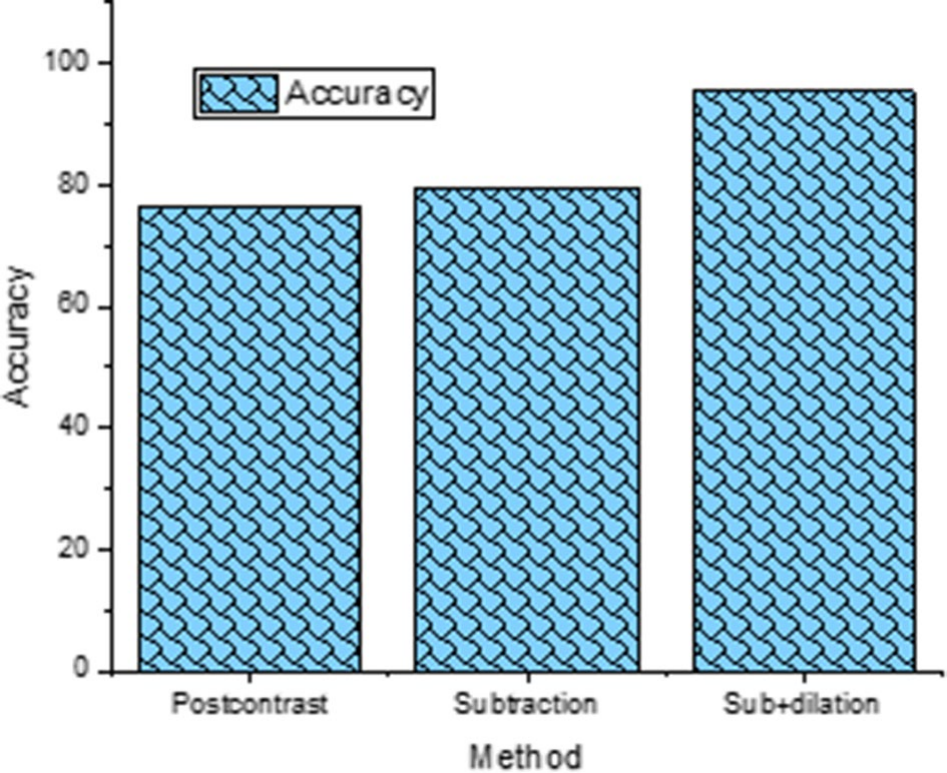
Accuracy for MRI texture analysis of tp1 + tp2 data + molecular subtypes for using image data of post-contrast MRI, subtraction of pre- and post-contrast MRI, and 5-pixel dilation of the subtracted images
